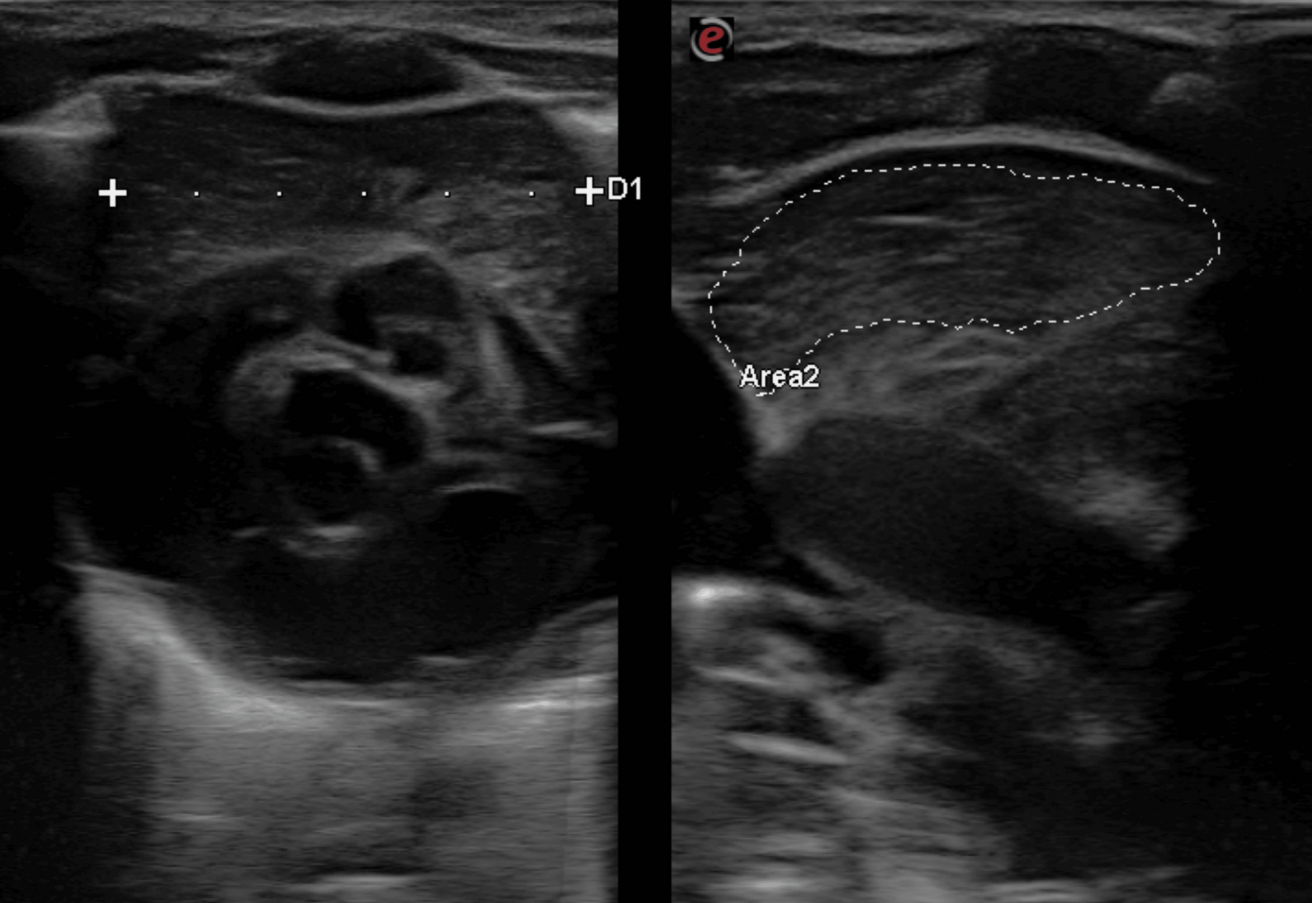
Ultrasonographic image of the thymus showing transverse diameter (D1) and sagittal area (Area2)
